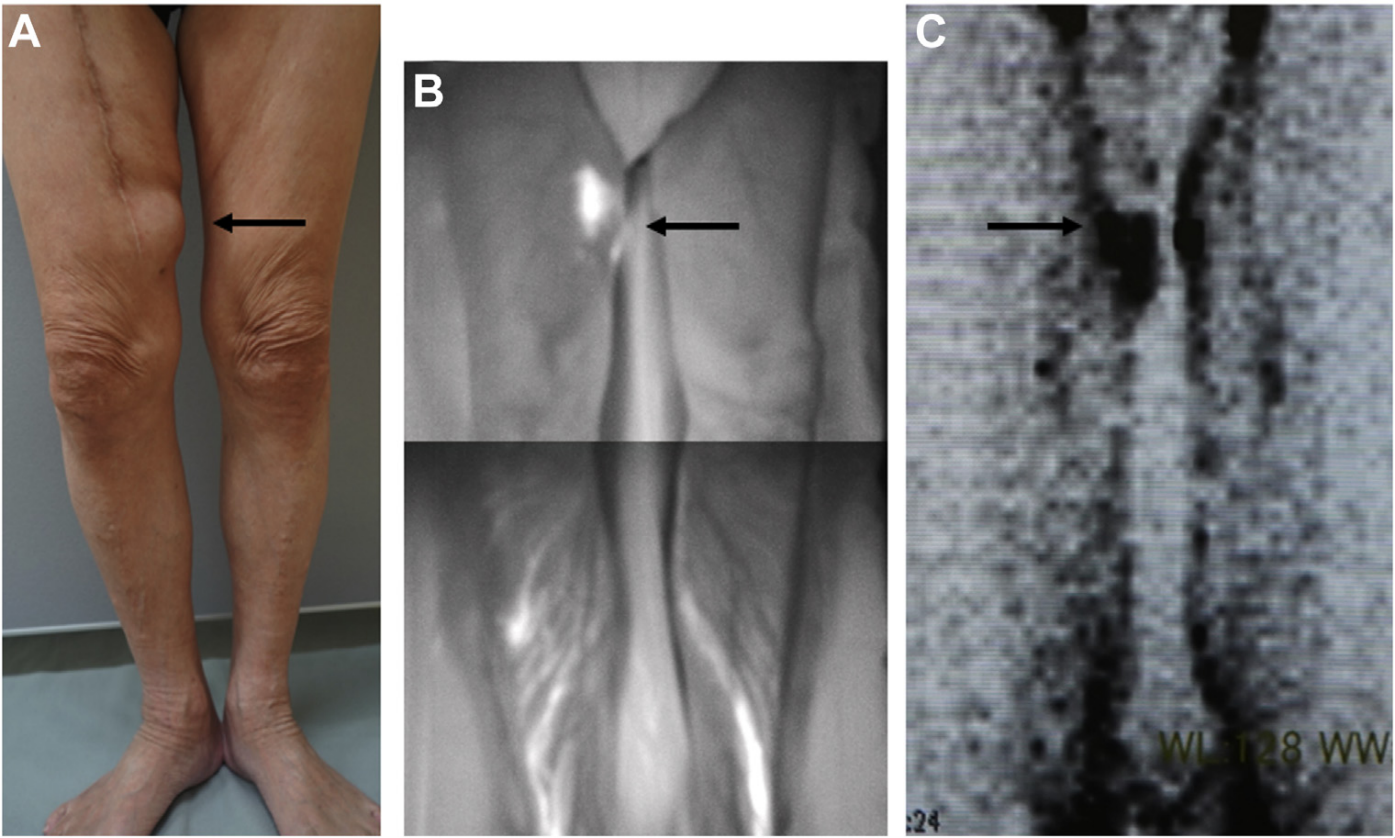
A, Photograph of the patient's lower extremities showing the lymphocele (*arrow*) in the medial side of the thigh along the scar where the great saphenous vein had been harvested. Indocyanine green lymphography (B) and lymphoscintigraphy (C) revealed pooling (*arrow*) at the site of the lymphocele.
